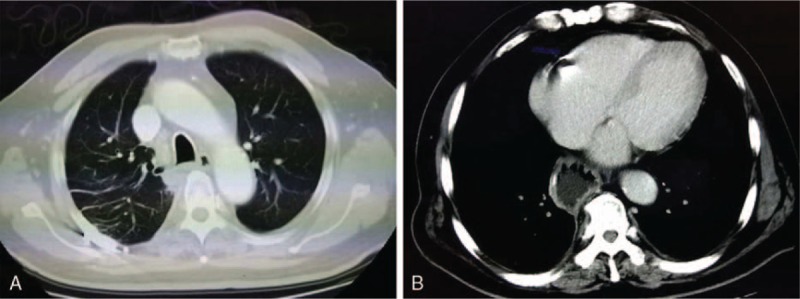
Computed tomography (CT) images excluded recurrence or metastasis 2 years after surgery.
